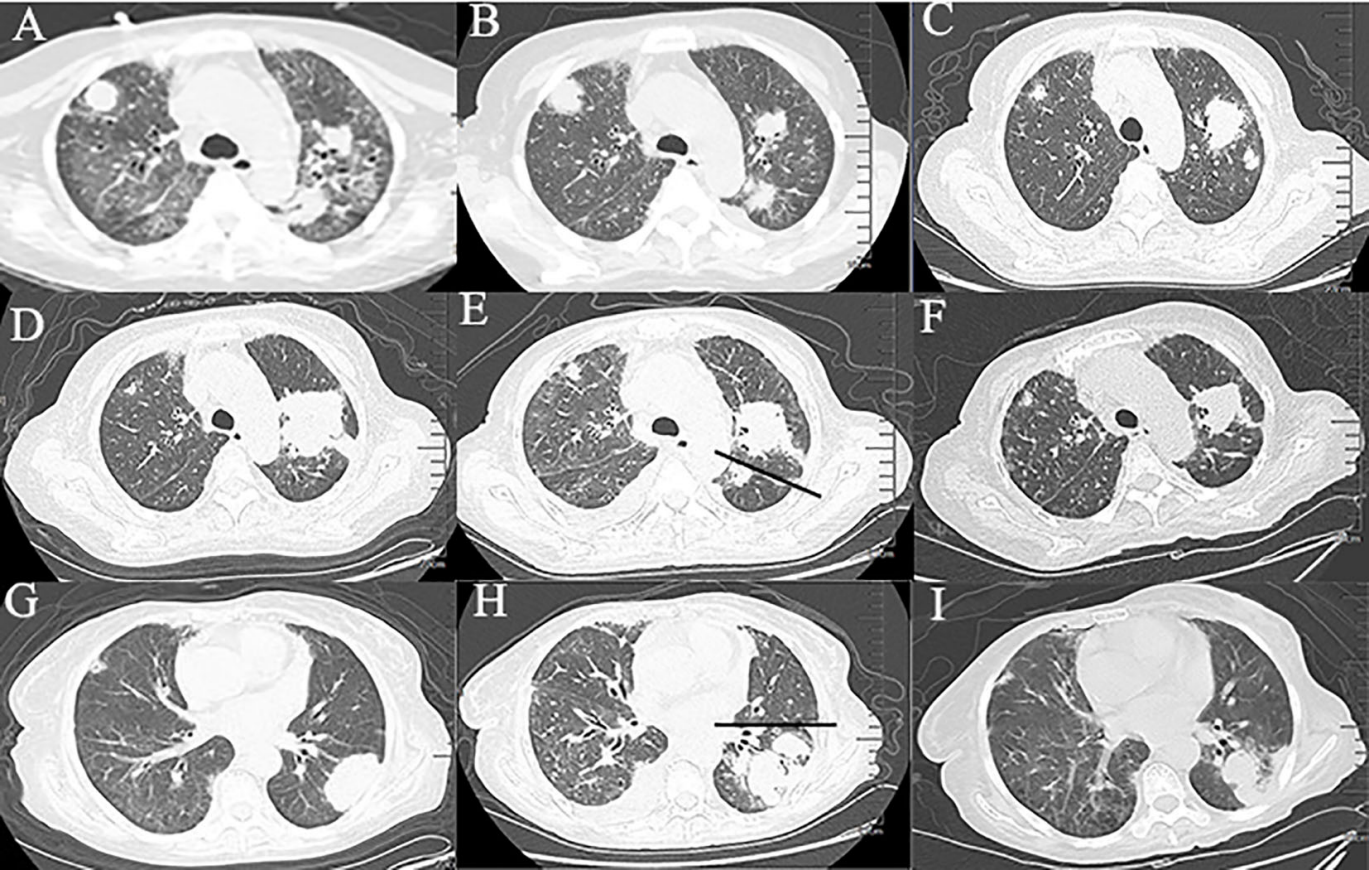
Chest computed tomography at different time periods. (A‒C) Changes of ground-glass opacities, patchy shadows, and multiple nodules in both lungs within 50 days. (D, E) Changes of masses in the upper lobe of the left lung within 20 days. (The straight line indicates the left interlobar fissure). (G, H) Changes of the masses in the lower lobe of the left lung within 20 days. (The straight line indicates the interlobar fissure). (F, I) Imaging findings after 2 courses of chemotherapy.
